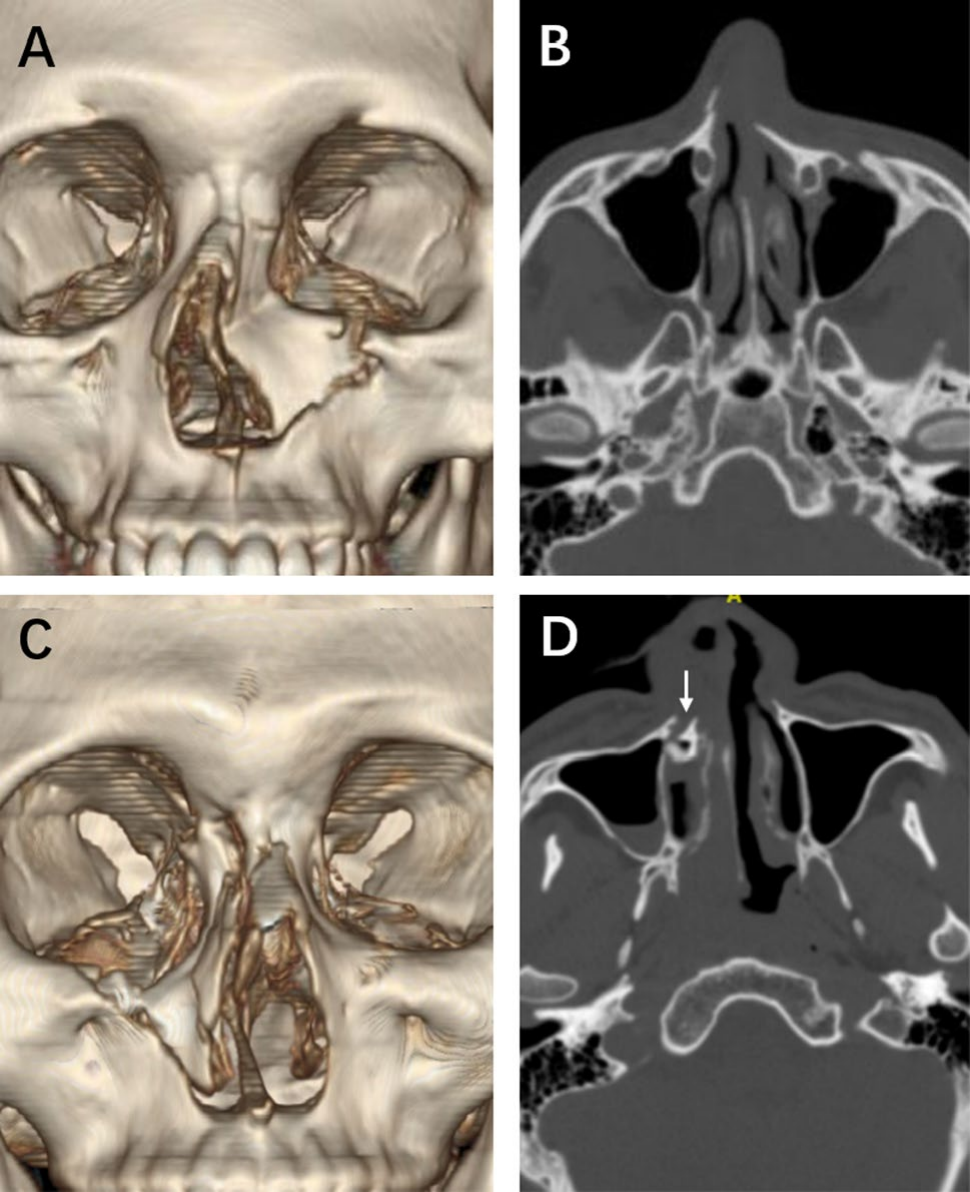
Type III fractures. CT images demonstrated a 17-year-old boy’s left pyriform buttress had a type III fracture, which caused the collapse of the ipsilateral nasal wall (A). The left nasal airway was completely blocked by the fractured bone fragment (B). Another young boy had comminuted type III fractures, with collapsing of the ipsilateral nasal wall and inferior turbinate (C, D). The right bony nasolacrimal canal was also fractured (D, white arrow)
